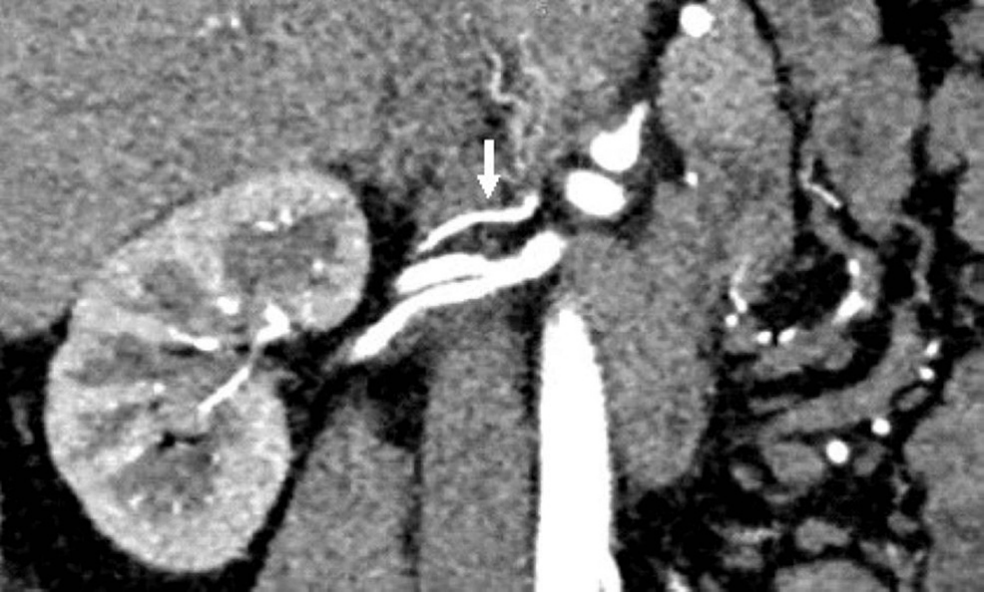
CT angiography shows prehilar early branching of right renal artery.
